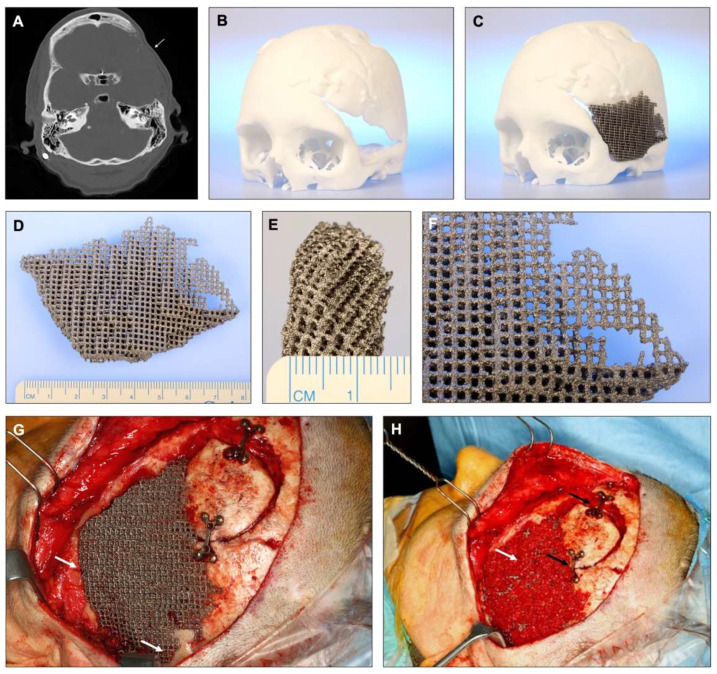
(A) Preoperative CT demonstrating the extensive bone defect (indicated by a white arrow) at the left frontotemporal region. (B) 3D-printed model of the patient’s skull, showing the triangular-shaped defect in the left frontotemporal region. (C) The computer-aided design and computer-aided manufacturing (CAD/CAM) titanium scaffold on the 3D-printed model of the patient’s skull. The CAD/CAM manufacturing allows the restoration of the bony outline in symmetry with the contralateral side. (D,E) Dimensions of the titanium scaffold. (F) Close up of the rough titanium surface showing an irregular structure. (G) Intraoperative situation immediately after reconstruction using the CAD/CAM titanium scaffold. Additional screws and a cerclage were used to fix the scaffold to the bone (white arrows). (H) titanium scaffold and overlaying iliac crest spongiosa (white arrow). A part of the defect could still be covered with autologous bone, fixed with two miniplates (black arrows).
